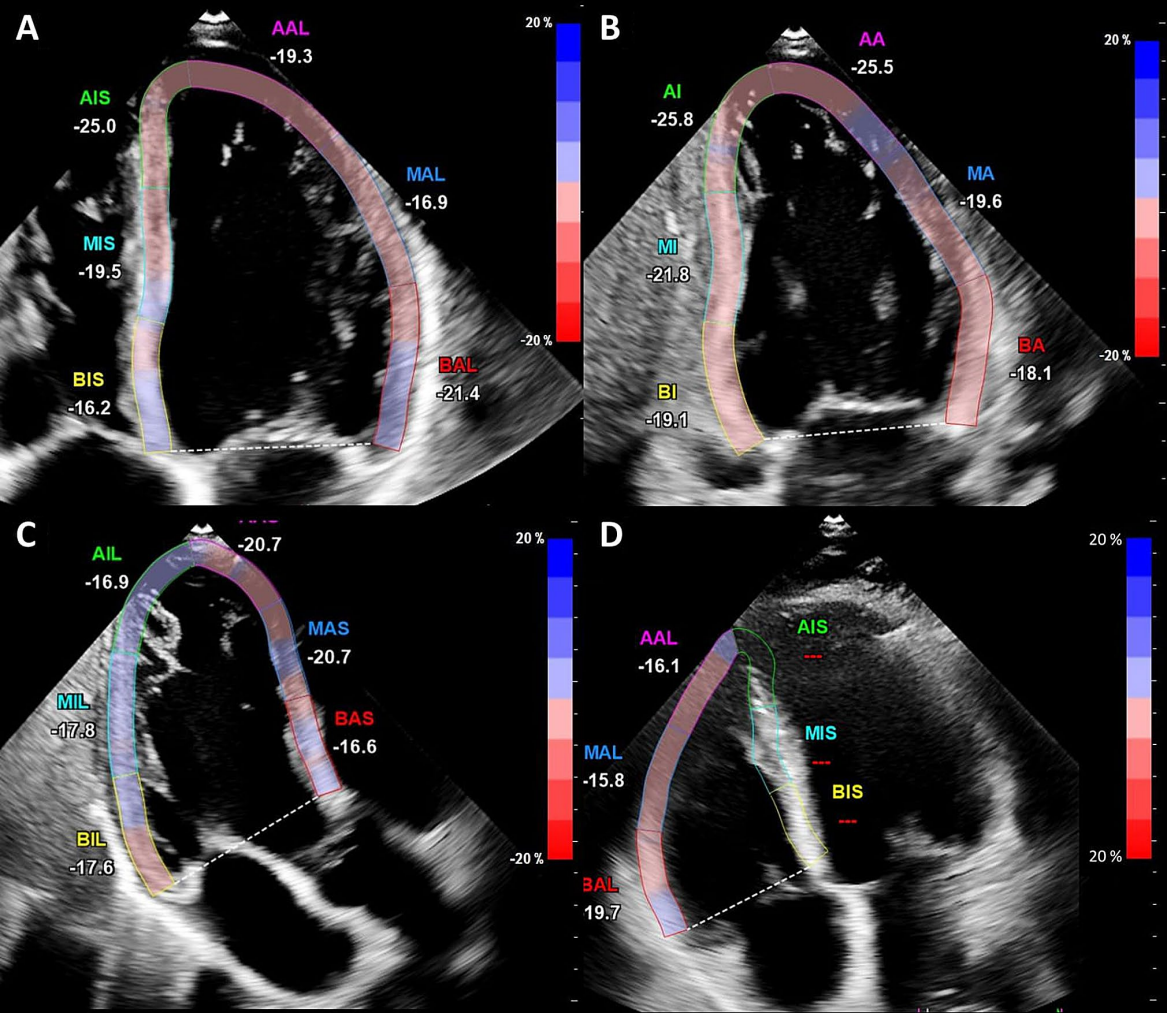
Strain analysis of the left and right ventricle Apical four a two b three chamber view c and a right ventricular focus view d were used for strain analysis. An end-diastolic frame was selected for the left ventricle (with the interventricular septum) and for the right ventricular free wall (without the interventricular septum), and the endocardial contour was manually tracked. The other frames were automatically tracked and corrected, if necessary. After verification the longitudinal strain was automatically calculated on average and regionally
